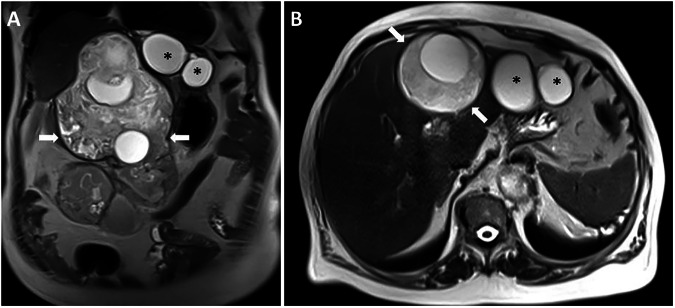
84-year-old female patient with epigastric pain underwent an abdominal MRI exam. Coronal (A) and axial (B) plane T2-weighted MRI images show a giant hydatid cyst (arrows) in the FL. Note small hydatid cysts (asterisks) in the left liver lobe
